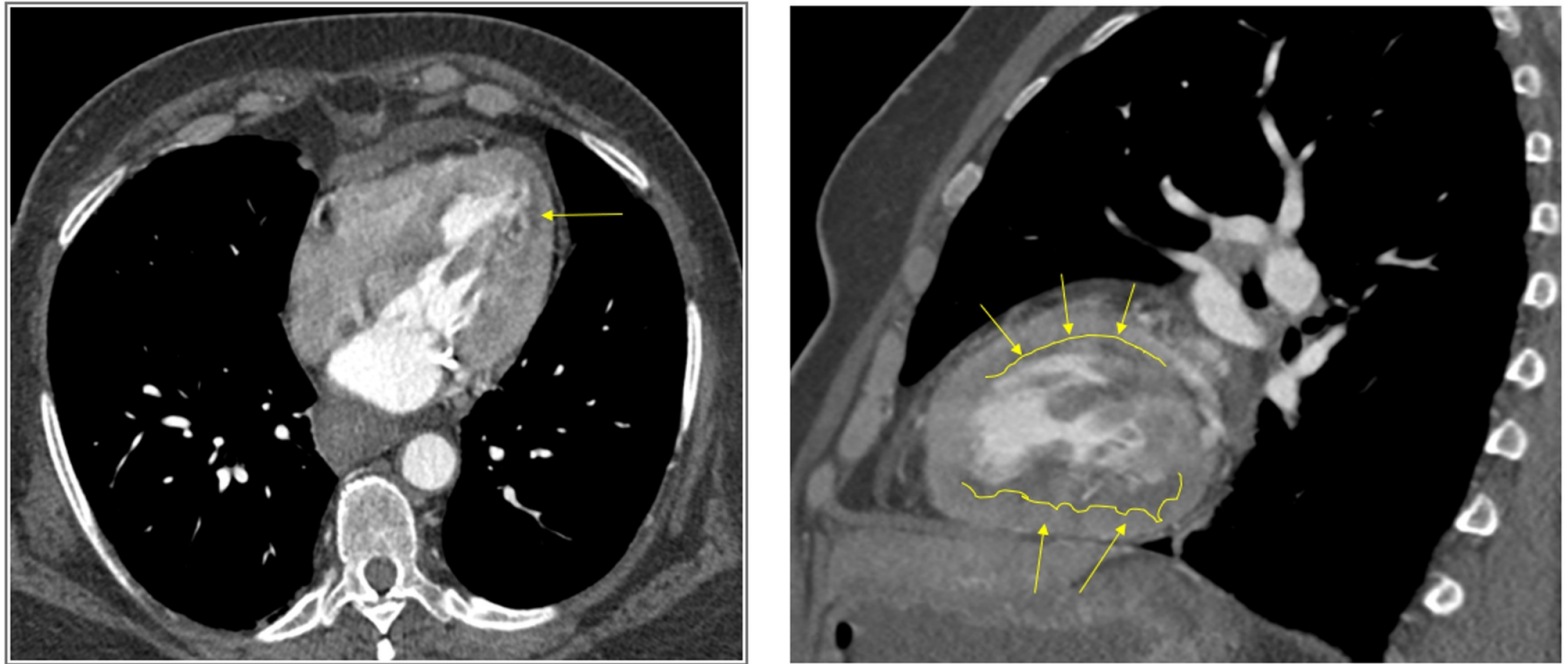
Cardiac MRI Subendocardial hypoperfusion, along with delayed enhancement in multiple vascular territories involving the left ventricular wall and papillary muscle in keeping with ischemia/infarction (yellow arrows).
